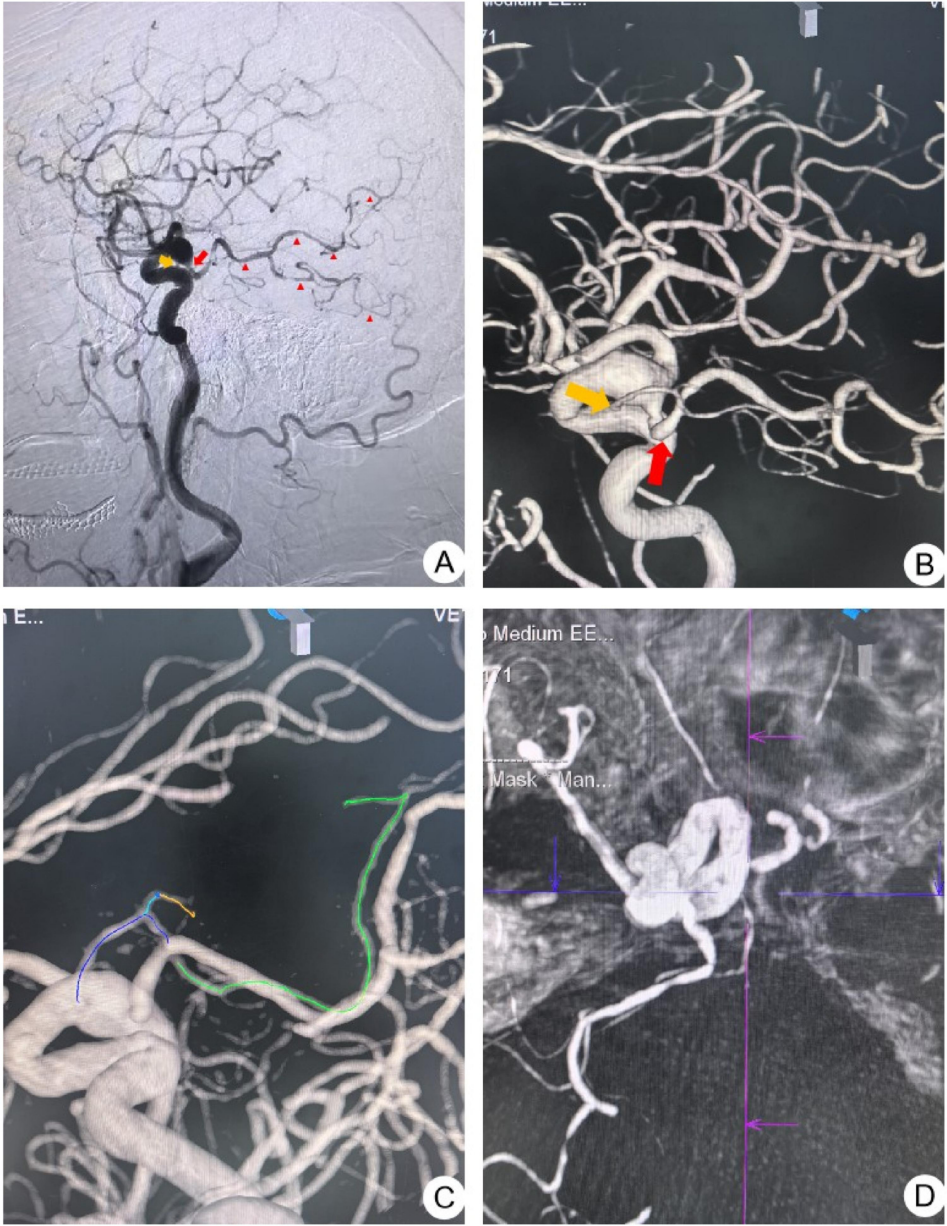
(A) Lateral digital subtraction angiography (DSA) of the right ICA shows fusiform dilatation of the C7 segment. The PCoA (red arrow) originates distally and supplies the frontotemporal region, with a small branch (AChA, yellow arrow) arising immediately proximal to its ostium. The area indicated by the red triangle represents the vascular territory of the PCoA. (B) Three-dimensional (3D) rotational angiogram delineates the sequential origins of the AChA (yellow arrow) and PCoA (red arrow). (C) A computer-automated tracing map depicts the entire course of the AChA: the purple line denotes the proximal segment of the AChA (cisternal segment, portion before entering the choroidal fissure), the green line represents the distal segment (plexal segment, portion after entering the choroidal fissure), and the blue and yellow lines indicate the anterior hippocampal artery. (D) Computed tomography angiography (CTA) confirms the origin of the AChA from the proximal segment of the ICA.
